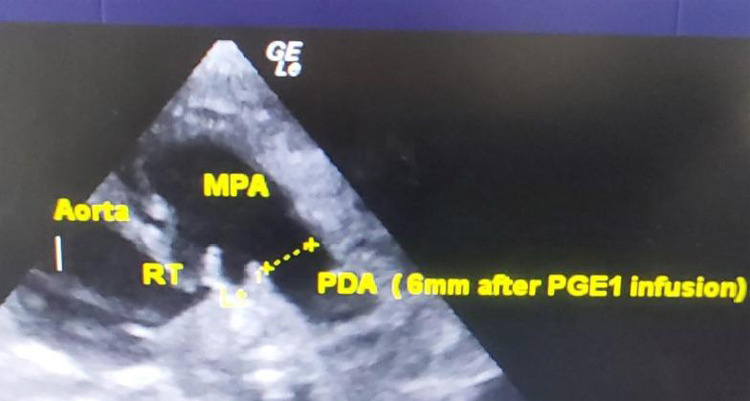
2D echocardiography parasternal short axis view showing hypoplastic aorta root, dilated MPA and large PDA after PGE1 infusion at 4 days of life.
